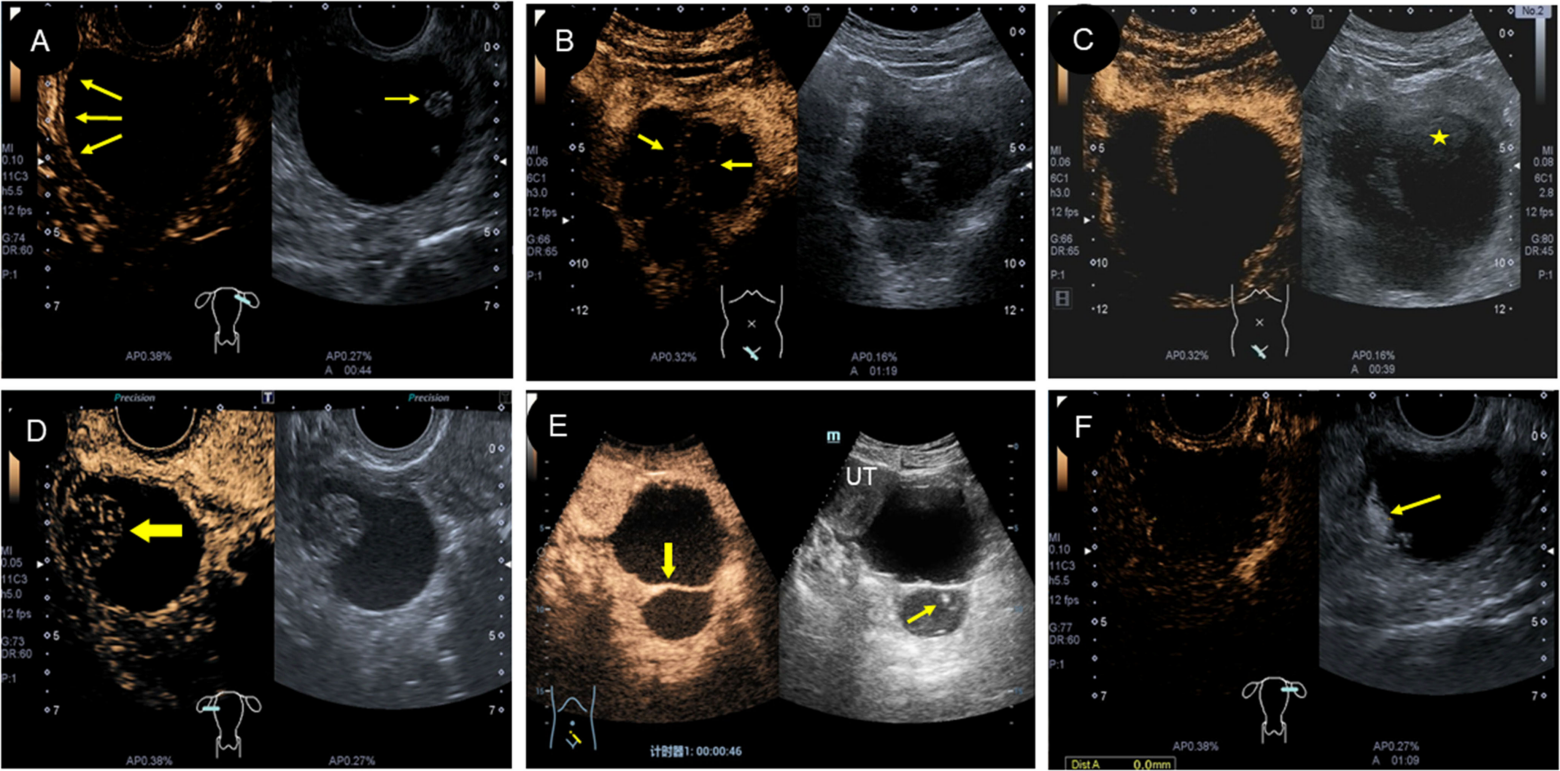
Characteristics of CEUS in different types of struma ovarii. (A) CEUS showed low enhancement of the cyst wall and papillary protrusion (arrow). (B) and (E) Different forms of septations in cyst were enhanced, but the light spot was unenhanced. (C) The “amniotic fat ball” (asterisk) in cyst showed no contrast agent perfusion on CEUS. (D) CEUS displayed that the solid area (arrow) was low enhanced in a cystic-solid-type SO. (F) CEUS showed that the irregular protrusion in cyst wall (arrow) was low enhanced, which was verified as a struma carcinoid pathologically. CEUS, contrast-enhanced ultrasound; SO, struma ovarii.
